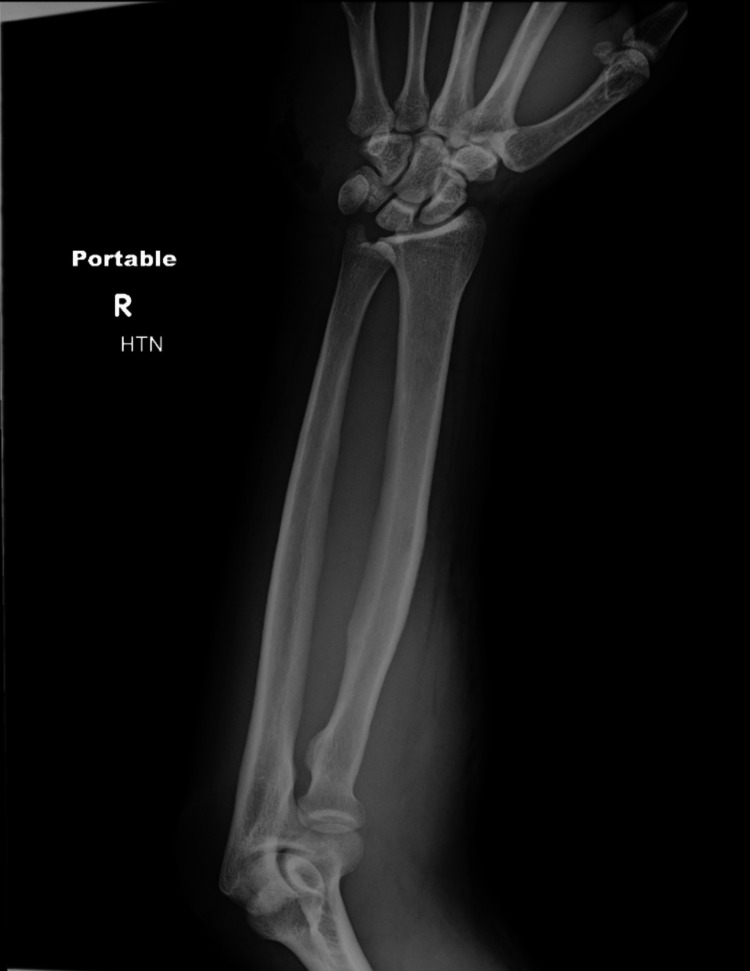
Frontal view of right forearm radiograph demonstrating soft tissue gas lucencies projecting over the medial hand.
